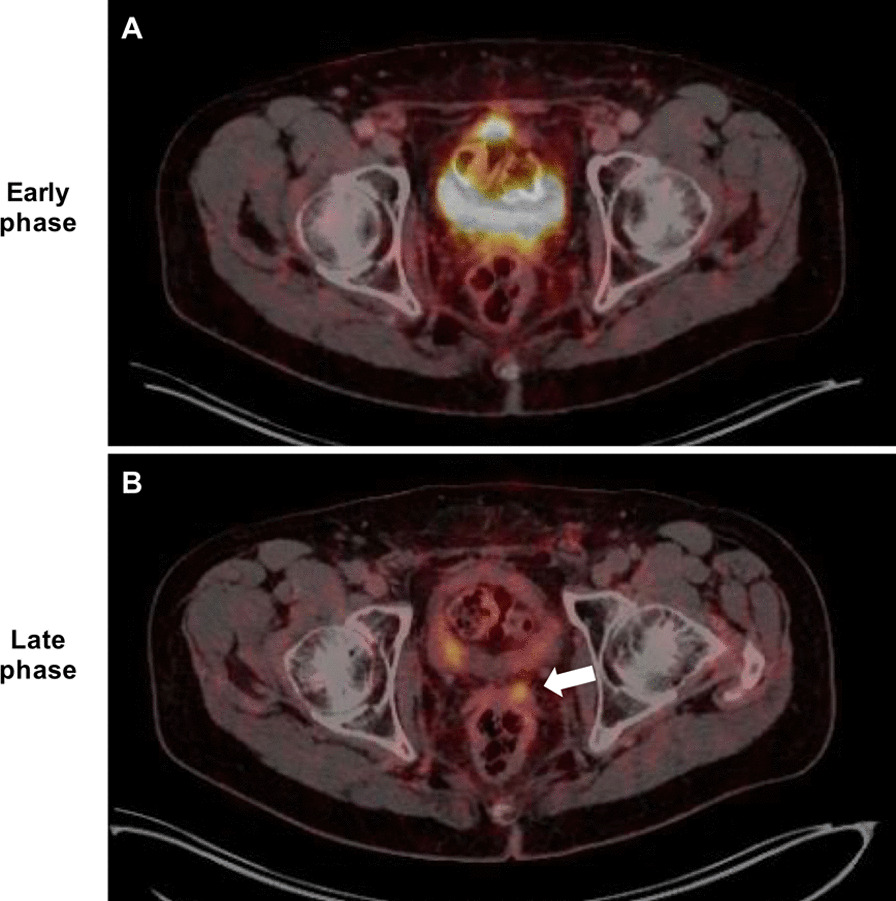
Example of a 72-year old patient with biochemical recurrence (PSA 1.76 ng/ml at the time of imaging). There was no tumorous lesion detectable on early-phase ^68^ Ga-PSMA PET/CT (A) because of obscuration due to the tracer in the urinary bladder. Late-phase ^68^ Ga-PSMA PET/CT performed after emptying of the urinary bladder (B) however, revealed the local recurrence in the seminal vesicles (white arrow)
